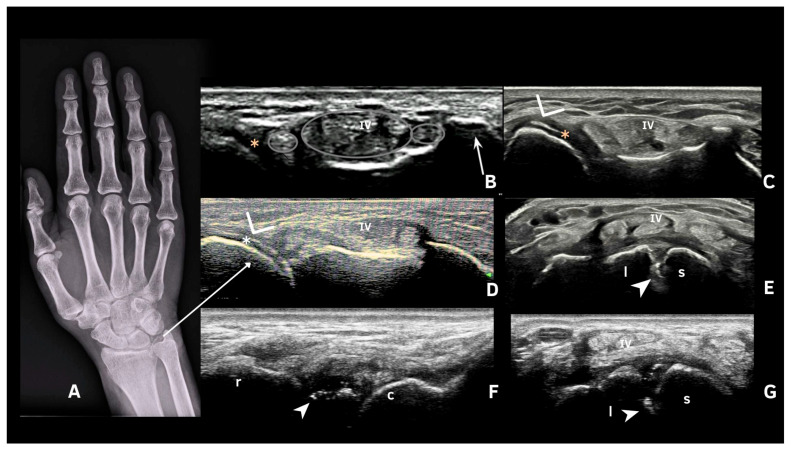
Wrist images. (A)—Radiograph showing CPP deposits in the distal radioulnar joint and triangular fibrocartilage (arrow). (B)—Transverse view at the level of the Lister’s tubercle of the radius (arrow) with the (iv) 4th extensor compartment. (*) Distal radioulnar joint. (C)—Linear deposition of calcium pyrophosphate in the distal radioulnar joint (*). (D)—Pseudo-double contour (*). (E)—Punctiform deposits (spots) in the scapho-lunate ligament (arrowhead); (l) lunate; (s) scaphoid. (F)—lLngitudinal scan of wrist scapho-lunate ligament with spots (arrowhead). (G)—Transverse scan of multiple spots on the scapho-lunate ligament (arrowhead). Source: personal files. c: capitate, IV: fourth compartment, l:lunate, r: radius, s: scaphoid.
